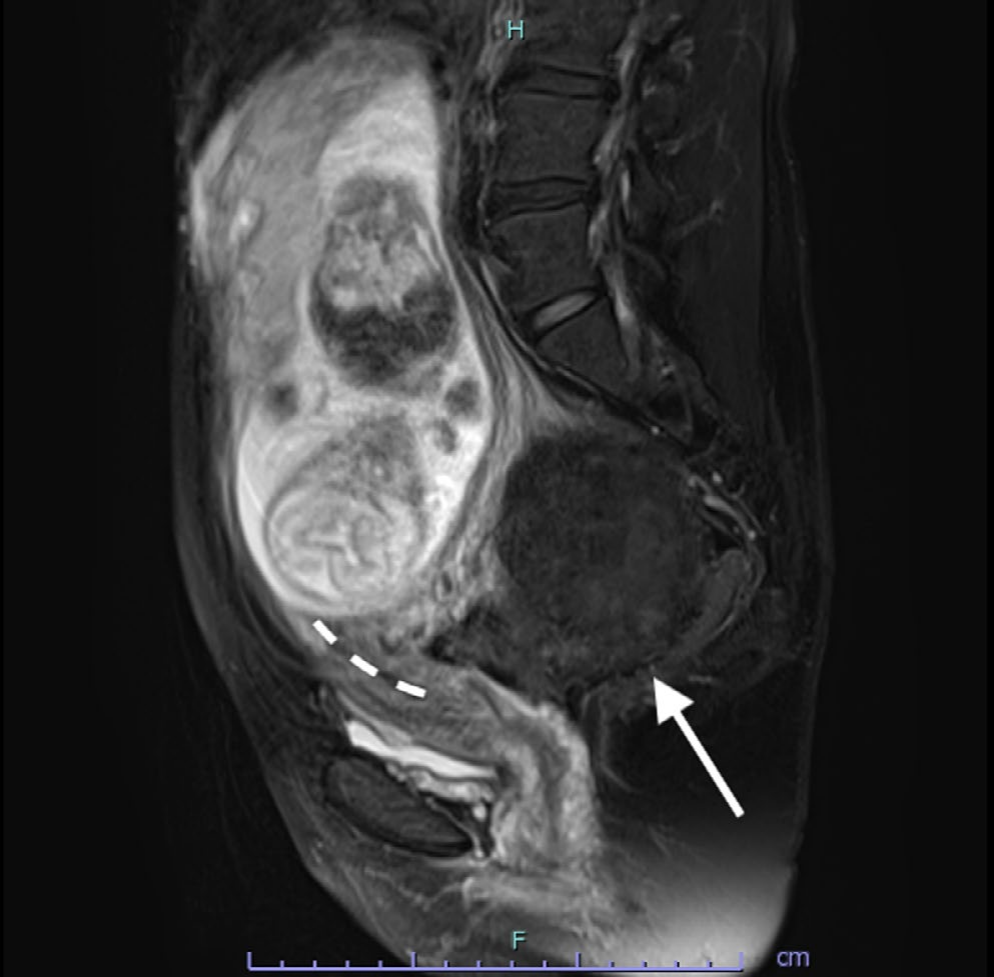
Magnetic resonance image at 19 wk of gestation (sagittal, T2‐weighted). The image shows an excessively stretched uterine cervix, one of the characteristic symptoms of the incarcerated gravid uterus. The uterus appears severely retroverted, and the uterine fibroid (white arrow) is visible in Douglas' pouch
